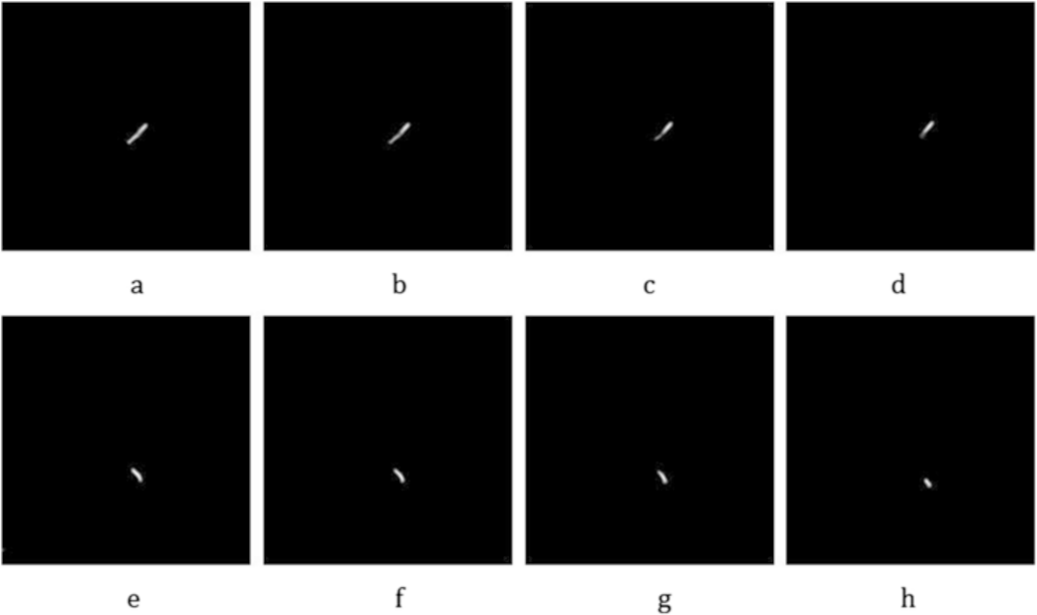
Coronary artery segmentation in each CTA slice using the proposed intersection tracking method. (a)-(d) Example of LAD segmentation. (e)-(h) Example of LCX segmentation.
